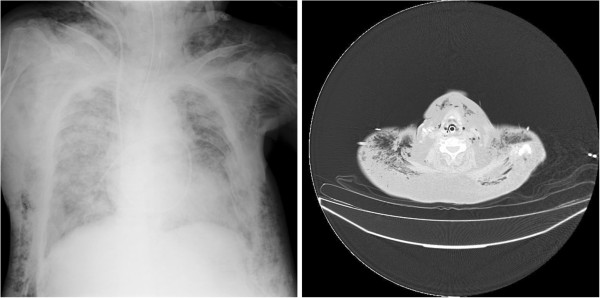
The chest X-ray and CT findings of the patient. Massive emphysema was observed in the neck, chest, and mediastinum.
